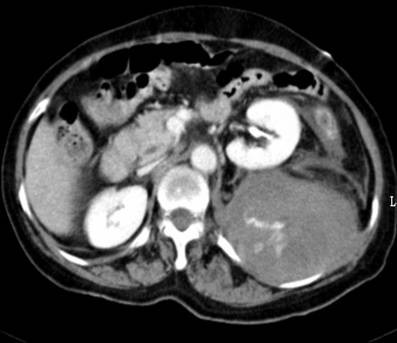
CT scan demonstrates left retroperitoneal hematoma with contrast medium extravasation.
